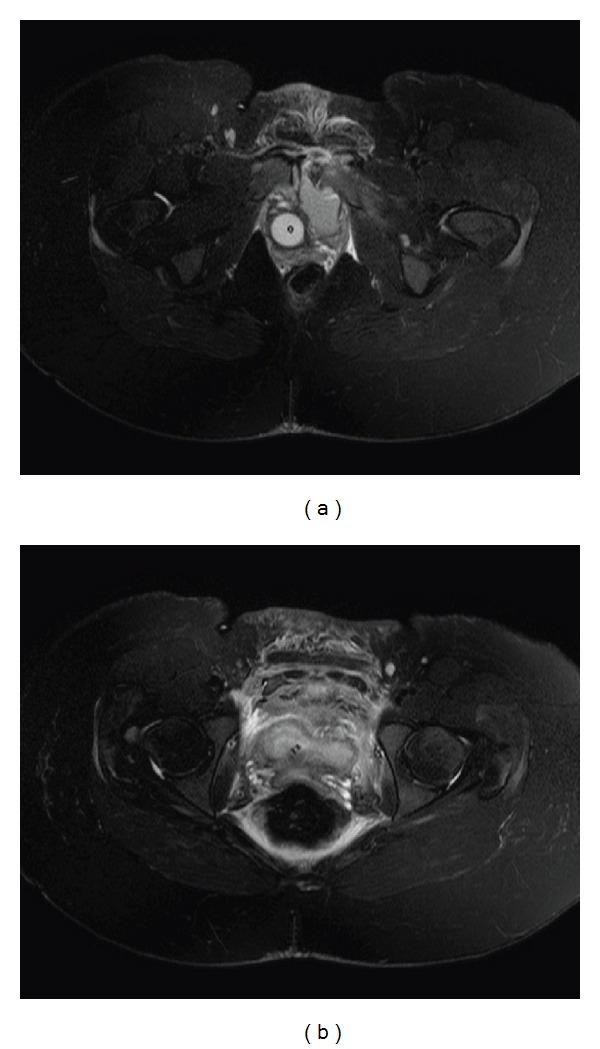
Axial T2-weighted images with fat-saturation (SPIR) are shown in (a) and (b). In addition, a hematoma around the symphysis pubis is shown, which extends to the small pelvis.
